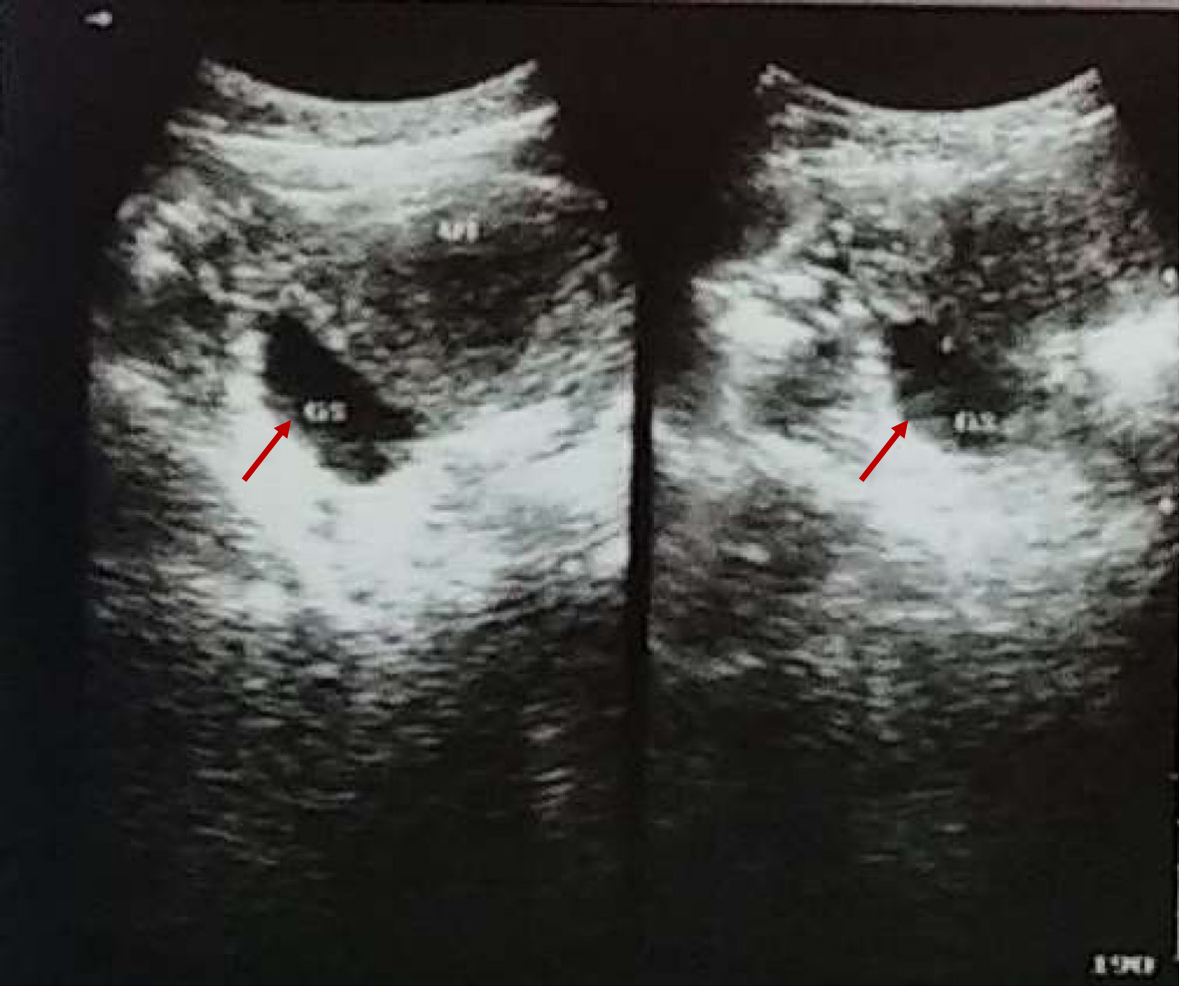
Pelvic ultrasound with arrows pointing to empty intrauterine gestational sac with mean sac diameter of 28 mm
